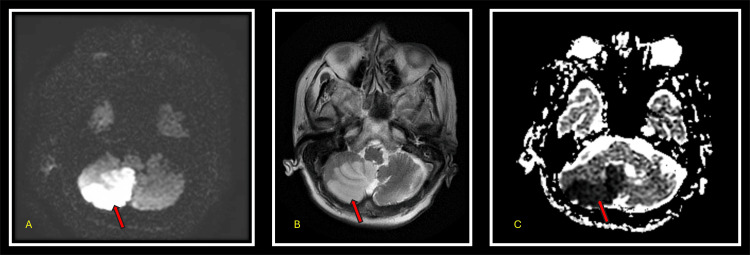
MRI brain showing right-sided ischemic cerebellar stroke. The red arrow points to the same lesion across different MRI sequences, the combination of which supports the diagnosis of an acute infarct. The lesion appears hyperintense on axial diffusion sequence (A) and axial T2 (B), and hypointense on apparent diffusion coefficient (C), consistent with diffusion restriction suggestive of acute infarct.
